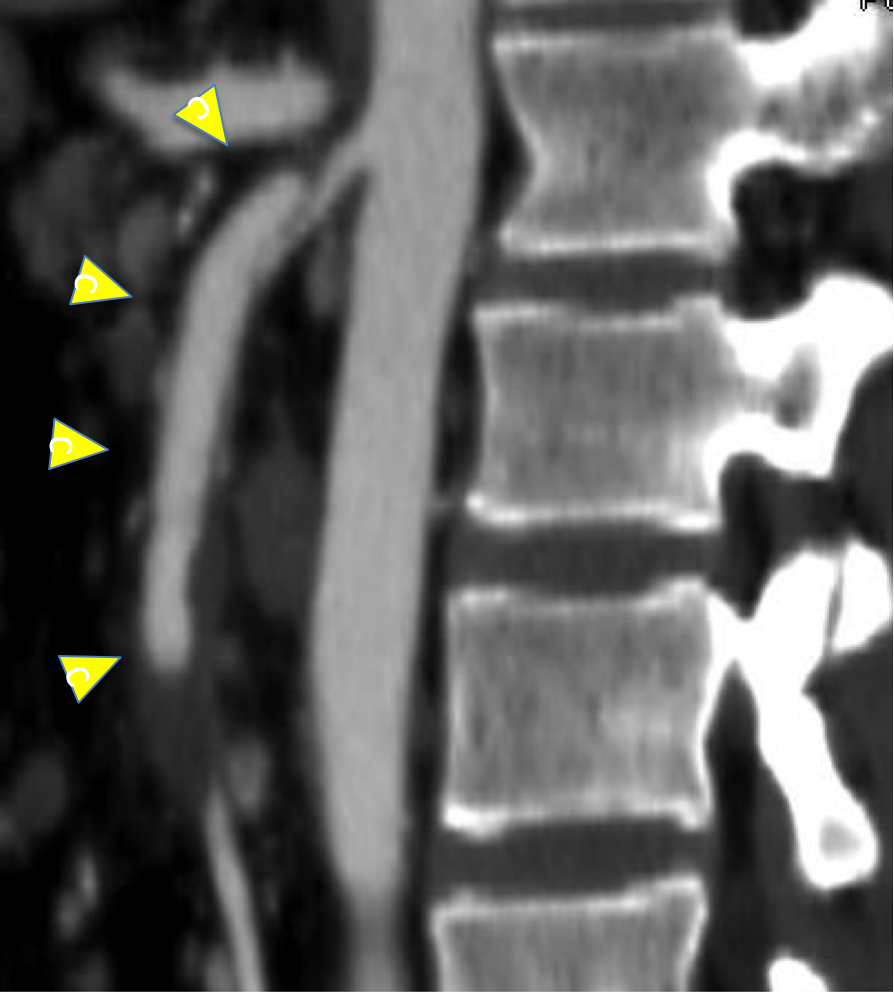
Computed tomography image 36 days after the symptom onset. Sagittal multiplanar reconstruction computed tomography showed that the false lumen was enhanced (arrowheads), but the true lumen was still compressed and thrombosed at the distal portion
